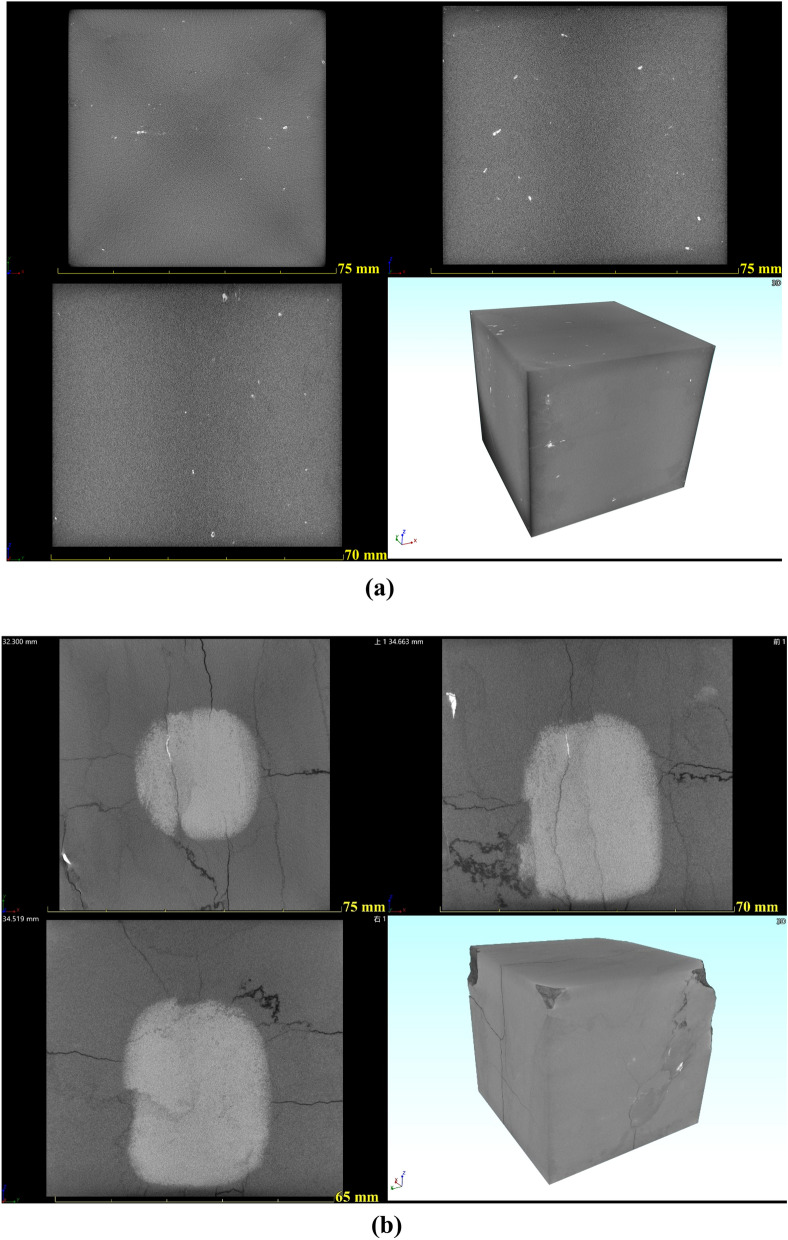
Four views of CT scan of limestone samples at typical temperatures: (a) 25 °C; (b) 1000 °C.
